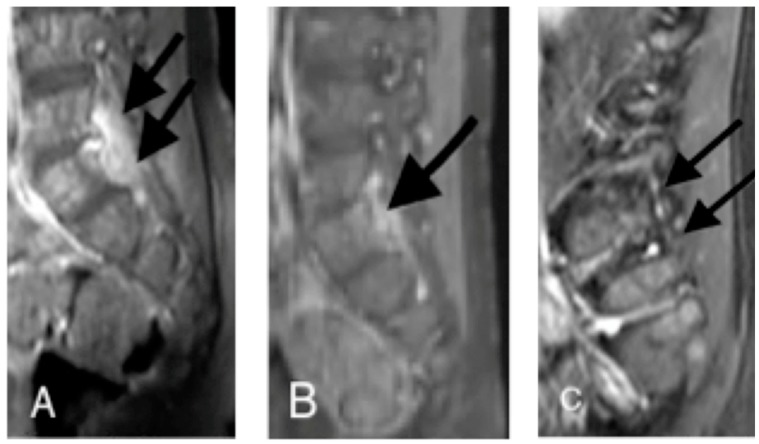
Lumbar spondylodiscitis with osteomyelitis, magnetic resonance imaging (MRI) of case 1. (A) Sagittal T1 post-enhancement image showing an increased signal of the annulus L4–L5, of the opposite end-plates of L4 and L5, of the adjacent soft tissues, and of an osteolytic area of the L5 pedicle (black arrow); (B,C) at the same level as A, after therapy (1 month and 4 months later, respectively), there is a progressive decrease in the pathological enhancement (black arrow), which has almost disappeared in C. Residual irregularities of the end-plates of L4 and L5 are evident.
